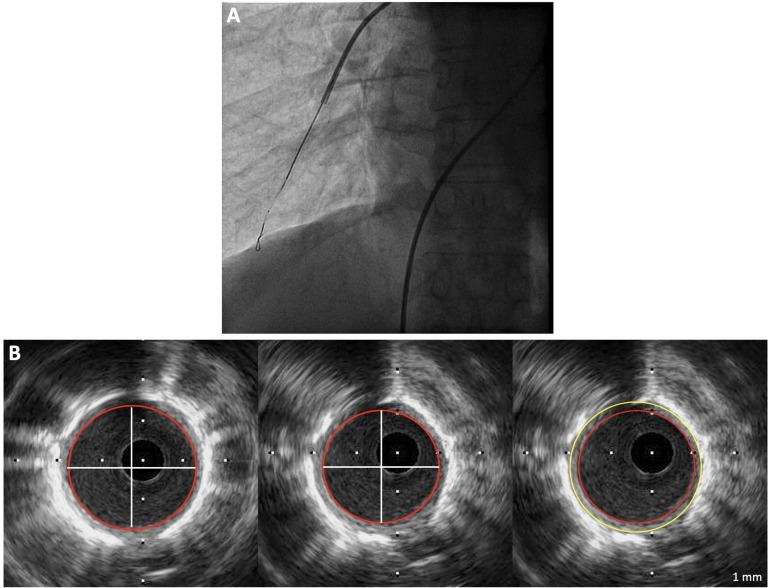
(A) Intravascular ultrasound probe positioning with X-Ray control. (B) Measurements of maximum systolic area, minimum diastolic area, and wall cross-sectional area in a pulmonary arterial hypertension patient (Diameter: 2.8 mm; pulsatility 31%; WCSA: 4.6 mm^2^; relative WCSA: 18%).
